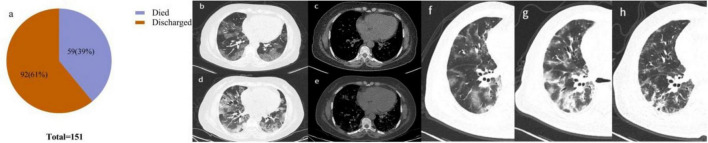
(a) Disease regression after treatment of patients with severe COVID-19 pneumonia. (b–e) Female, 69 years old, with fever, cough and chest tightness for 6 days. (b,c) 2022-12-29 CT, multiple nodular, lattice-like and patchy hyperdense shadows in both lungs with slight local pleural thickening; (d,e) On 2023-01-03 CT, the lesion was more extensive than before, with increased density, localized densities and localized thickening of the pleura bilaterally. (f–h) Male, 67 years old, fever with cough for 10 days. (f) 2022-12-25 CT, multiple patchy, banded ground glass density shadows are seen in the lung field with a predominant subpleural and peribronchial vascular bundle distribution with blurred, partially dense margins. (g) 2023-01-01 CT, most lesions are more extensive and denser than before, with overall progression from before. (h) 2023-01-09 CT, partially less extensive and less dense than before, overall better than before.
